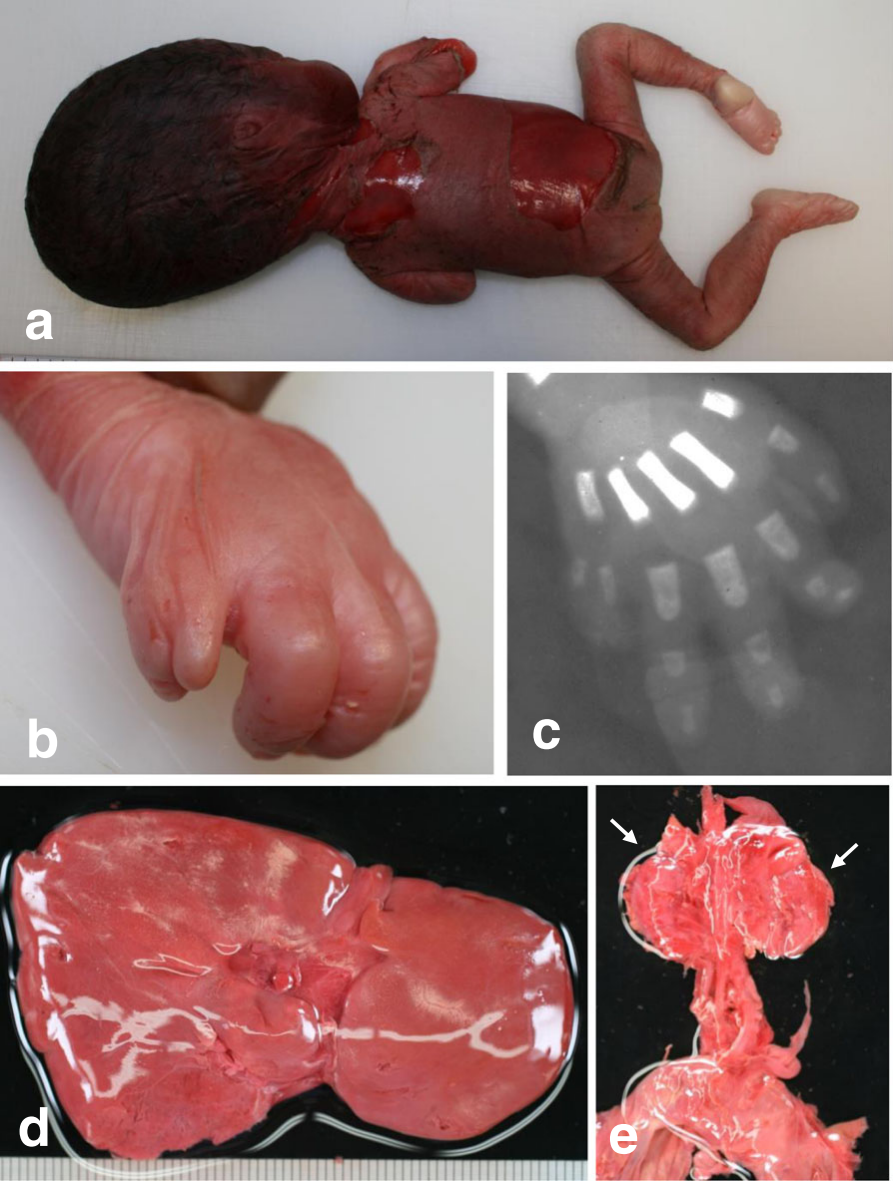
Macroscopic findings at autopsy. The stillborn had a proportionally unbalanced, large head with acrocephaly (a). Postaxial polysyndactyly of the right hand was noted (b) and (c, photography by soft X-ray). While the shape and size of the liver were normal, the gallbladder was nonexistent at the hepatic portal section (d, hatched area). Hypoplasia of both kidneys (e) was noted (arrows)
